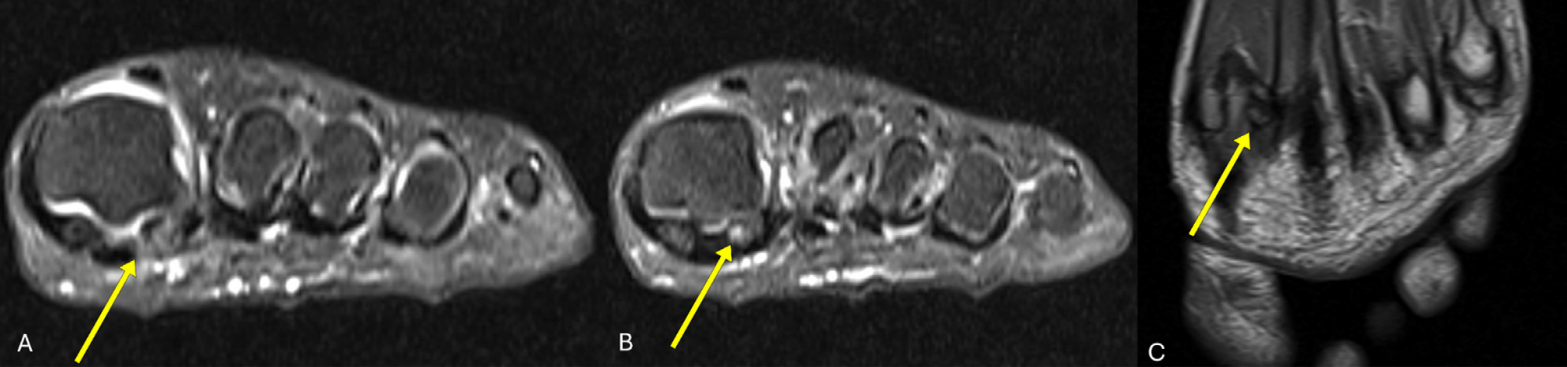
(A) and (B) Axial fat-suppressed proton density (PD-FS) images and (C) coronal T1-weighted image of the left forefoot. Yellow arrows indicate increased signal intensity of the lateral hallucial sesamoid on PD-FS, consistent with bone marrow edema and compatible with AVN. The T1-weighted image demonstrates corresponding hypointensity.
